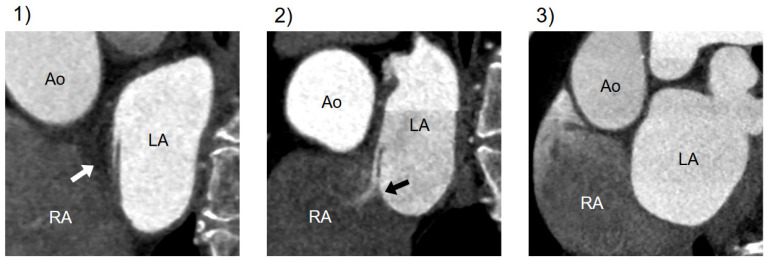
Variations in CT imaging on the atrial septum. Cardiac CT findings of the atrial septum on a short-axis view through the atria: (1) CLA without contrast jet flow; (2) CLA with jet flow toward RA; (3) normal interatrial septum without a visible channel. CT, computed tomography; Ao, aorta; LA, left atrium; RA, right atrium; CLA, channel-like appearance.
